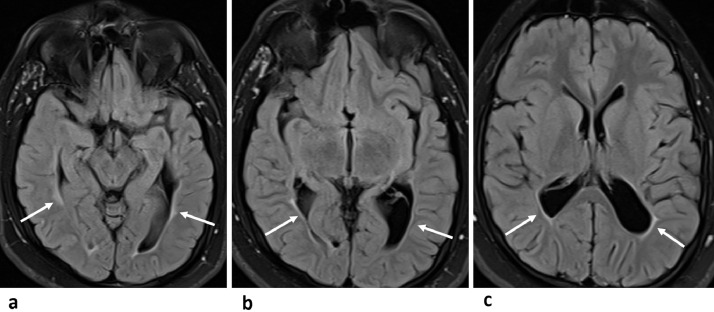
(A-C) Axial FLAIR-T2W images from inferior to showing evidence of posterior predominant volume loss of deep periventricular white matter, with ex-vacuo dilatation of the atria-trigones, temporal, and occipital horns of the lateral ventricles on both sides with irregular wavy outline, and peri-ventricular bright signal intensity gliotic changes abutting the ependymal surface (arrows in A-C).
